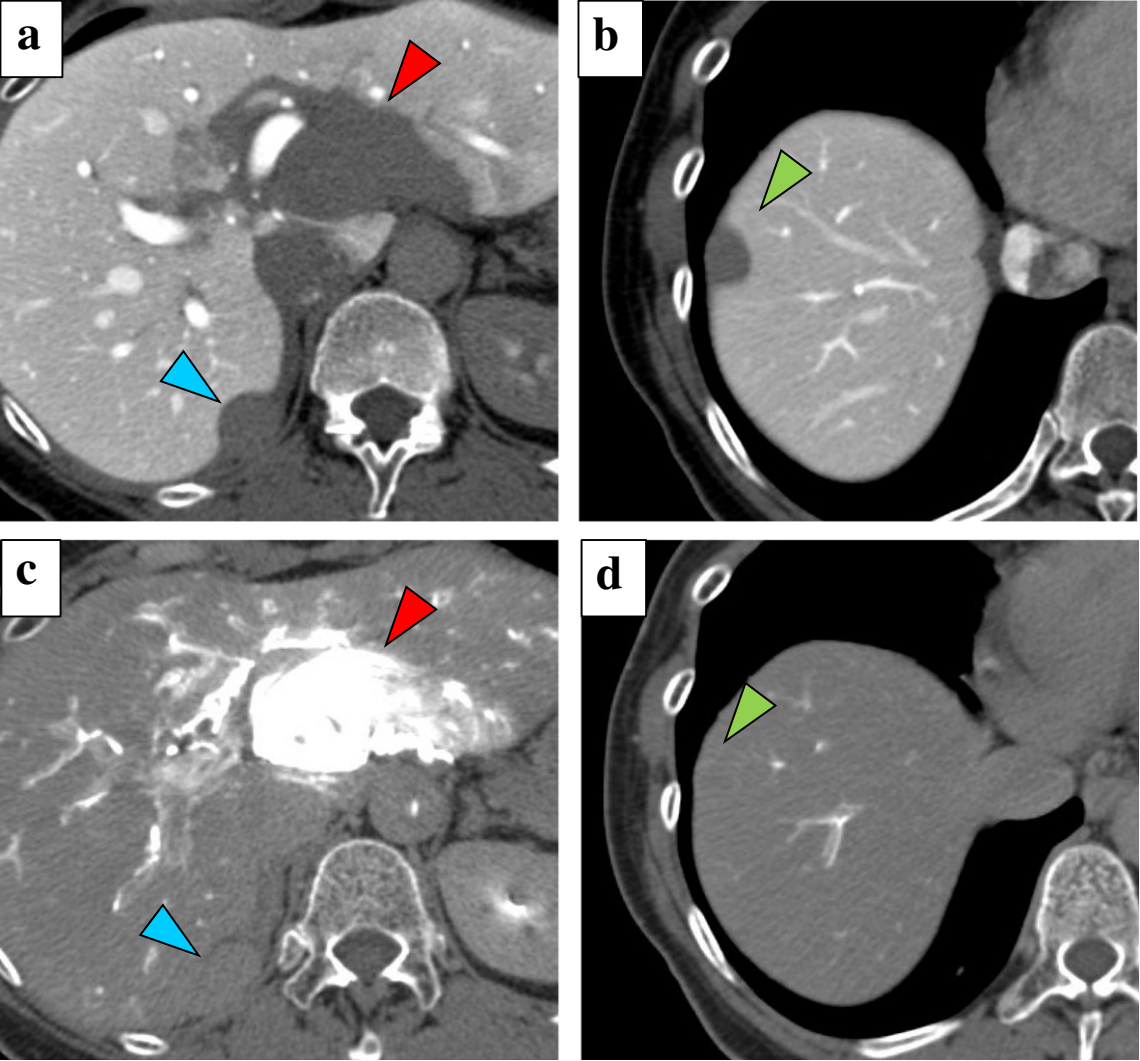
Computed tomography (CT) during arterial portography showed enhancement in none of the tumors (a, b), but CT during hepatic arteriography revealed strong enhancement in the tumor in the lateral segment. In contrast, the remaining three tumors showed no enhancement (c, d) (*red arrow*, the tumor in the lateral segment; *blue arrow*, the tumor in segment 7; *green arrow*, the tumor in the in segment 8)
